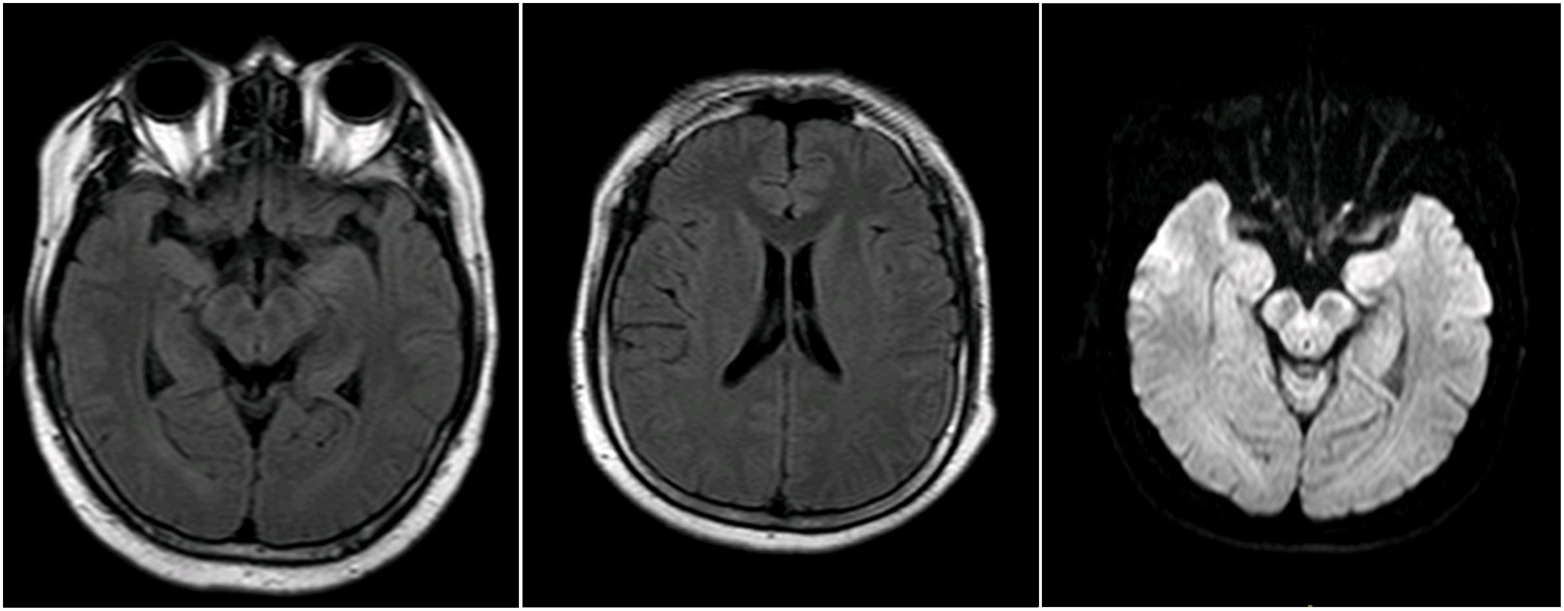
Brain MRI images of the patient.
